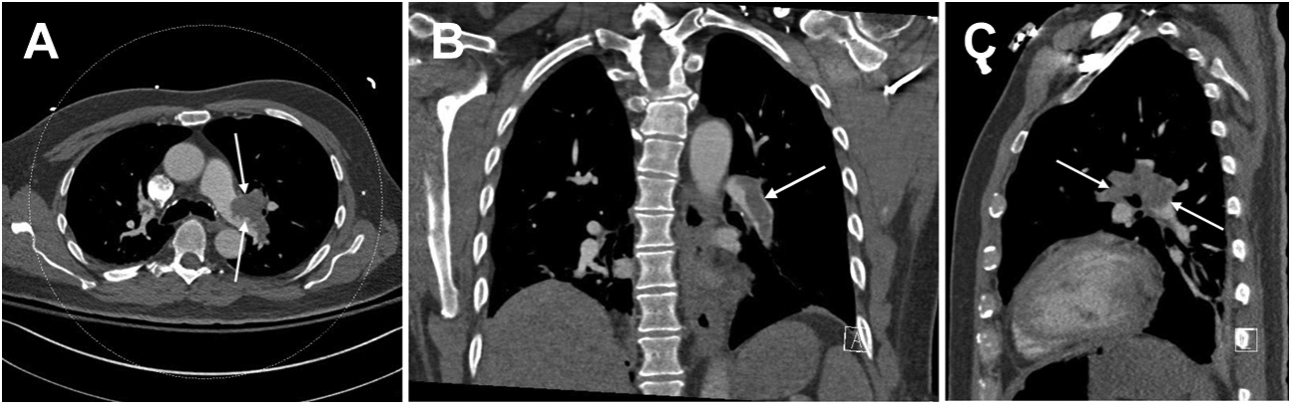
Computed Tomography Pulmonary Angiography of Central Pulmonary Embolism Computed tomography pulmonary angiographic view demonstrating near-occlusive left pulmonary artery thrombus in (A) axial, (B) coronal, and (C) sagittal multiplanar reformats (arrows).
